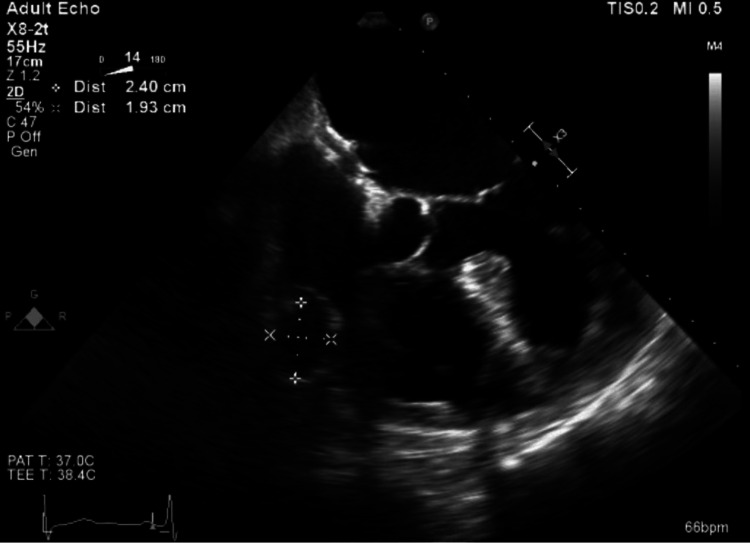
Echocardiogram showing a giant right coronary artery aneurysm
